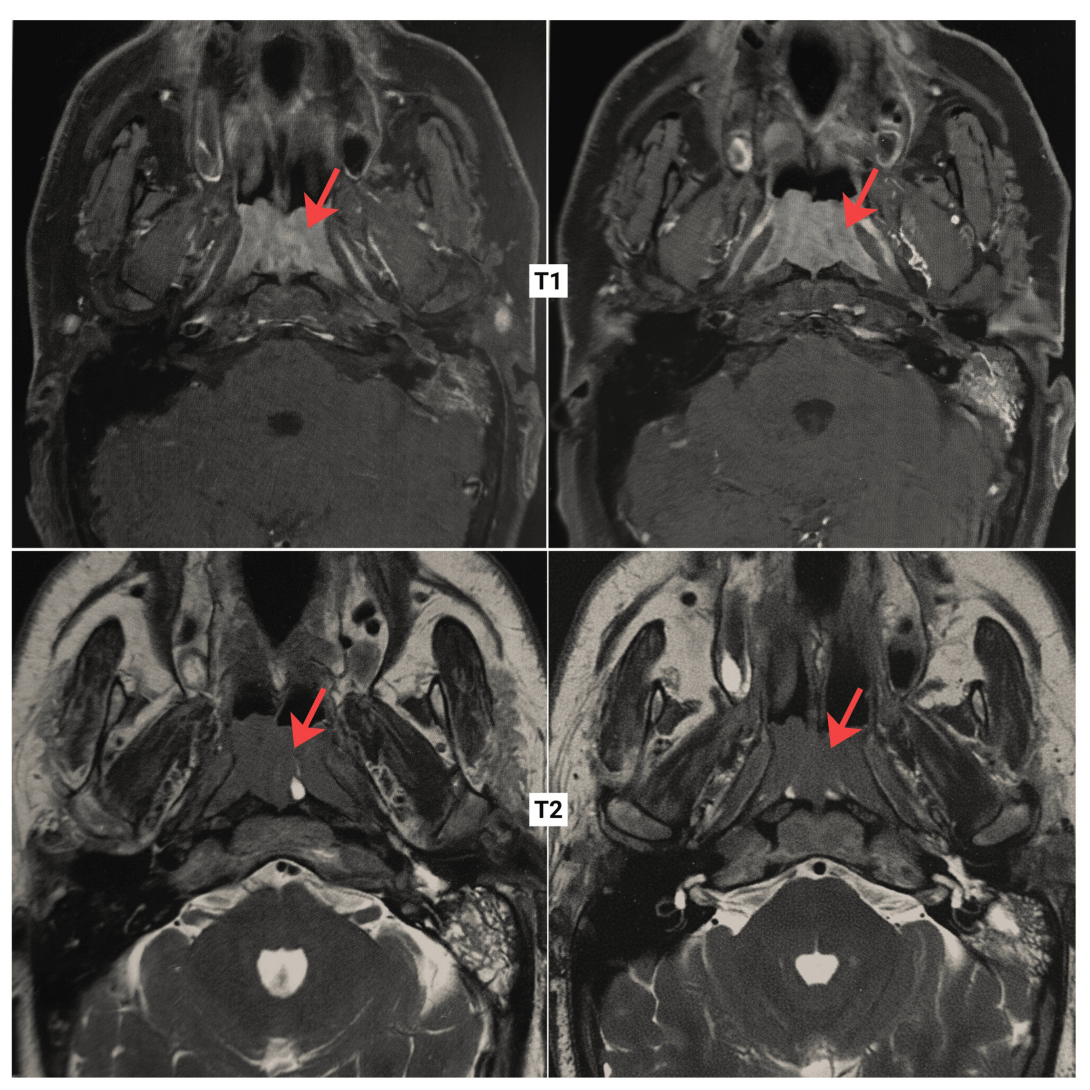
Axial T1- and T2-weighted MRI showing nasopharyngeal involvement in chronic lymphocytic leukemia. Topographic assessment showed that the mass was laterally limited by the nasopharyngeal wall without extension into the lateral pharyngeal spaces. Anteriorly, it reached the choanae, posteriorly it was in contact with the prevertebral muscles without significant invasion, superiorly it abutted the sphenoid sinus with no evidence of bony or intracranial extension, and inferiorly it extended to the pharyngeal and hypopharyngeal walls as a semi-circumferential mucosal thickening. Intense contrast enhancement was also observed at the tonsillar region, continuing with the mucosal thickening and extending to the lingual tonsils. The left mastoid cells and middle ear were opacified. Overall, the imaging findings were consistent with a localized nasopharyngeal lesion.
